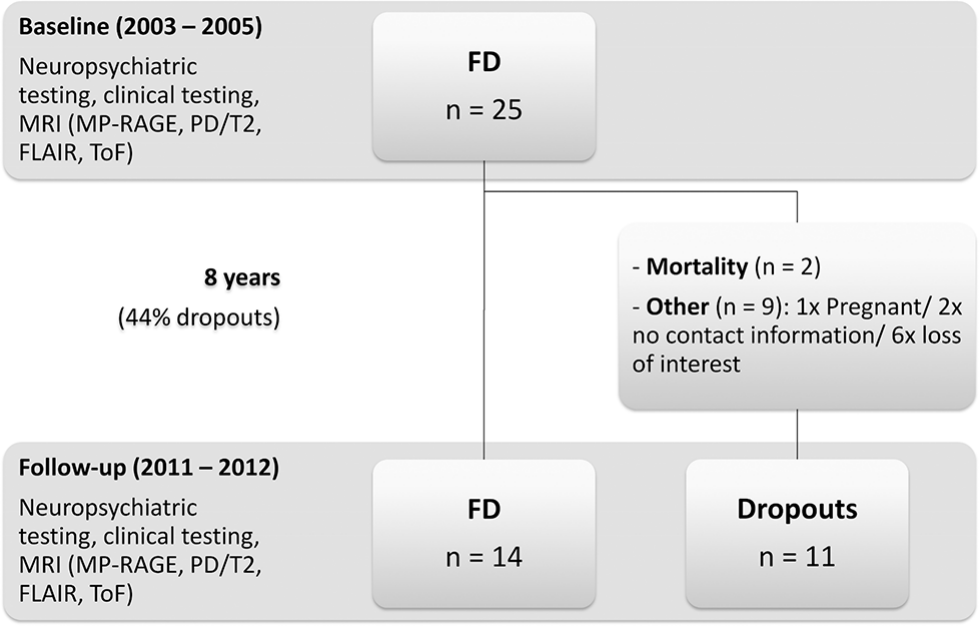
Study design of the longitudinal assessment. FD = Fabry disease; MRI = Magnetic resonance imaging.
